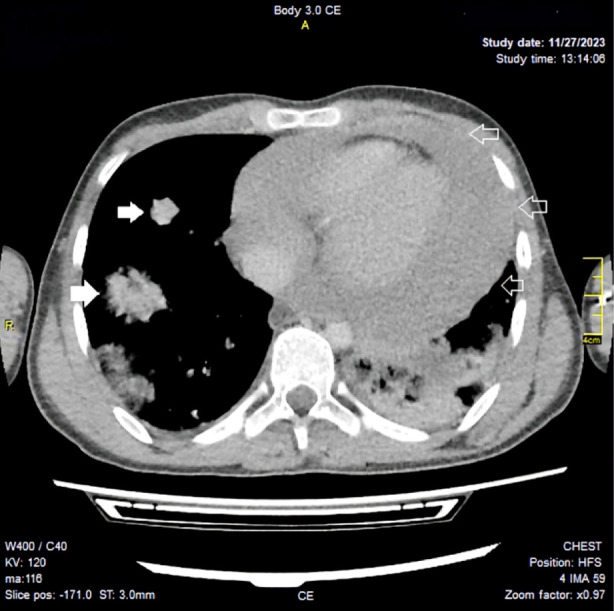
The CT chest: a) infiltrates in the lung fields (solid arrows); b) pericardial effusion (arrow outlines).
